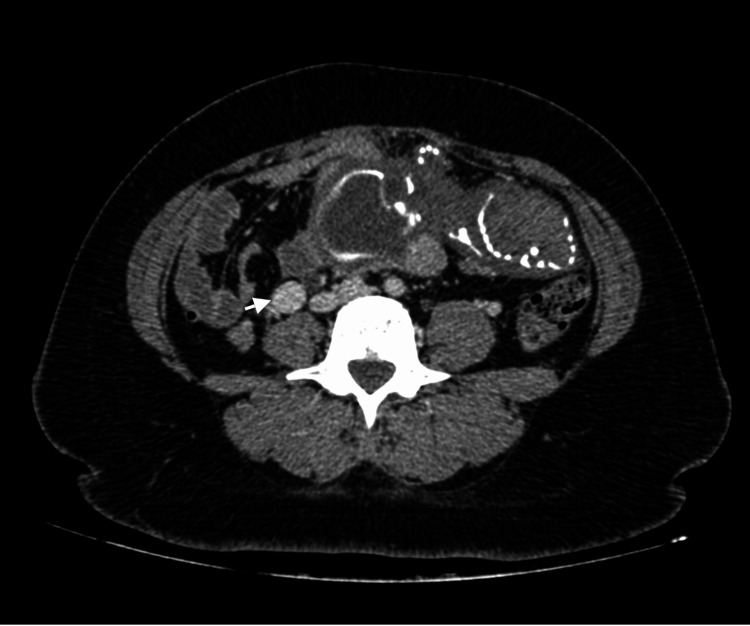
Axial, venous phase post-contrast CT, through the pelvis, showing engorged, tortuous right ovarian vein (arrow).
